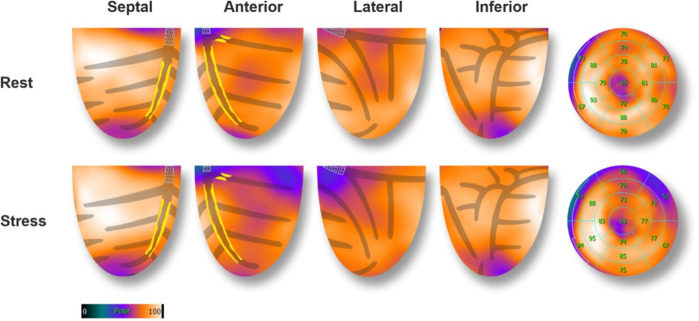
82-Rubidium PET-CT scan 23 days after the PCI of the MT, proximal LAD, and CX arteries. Compared to the baseline exam, images at rest showed a significant improvement in myocardial perfusion after the revascularization. Images during pharmacological stress showed an 8% severe defect in perfusion of the anterolateral basal segment, corresponding to the territory of the 1st diagonal artery (70% focal stenosis of the proximal part). A PCI was subsequently performed (Figure 3).
